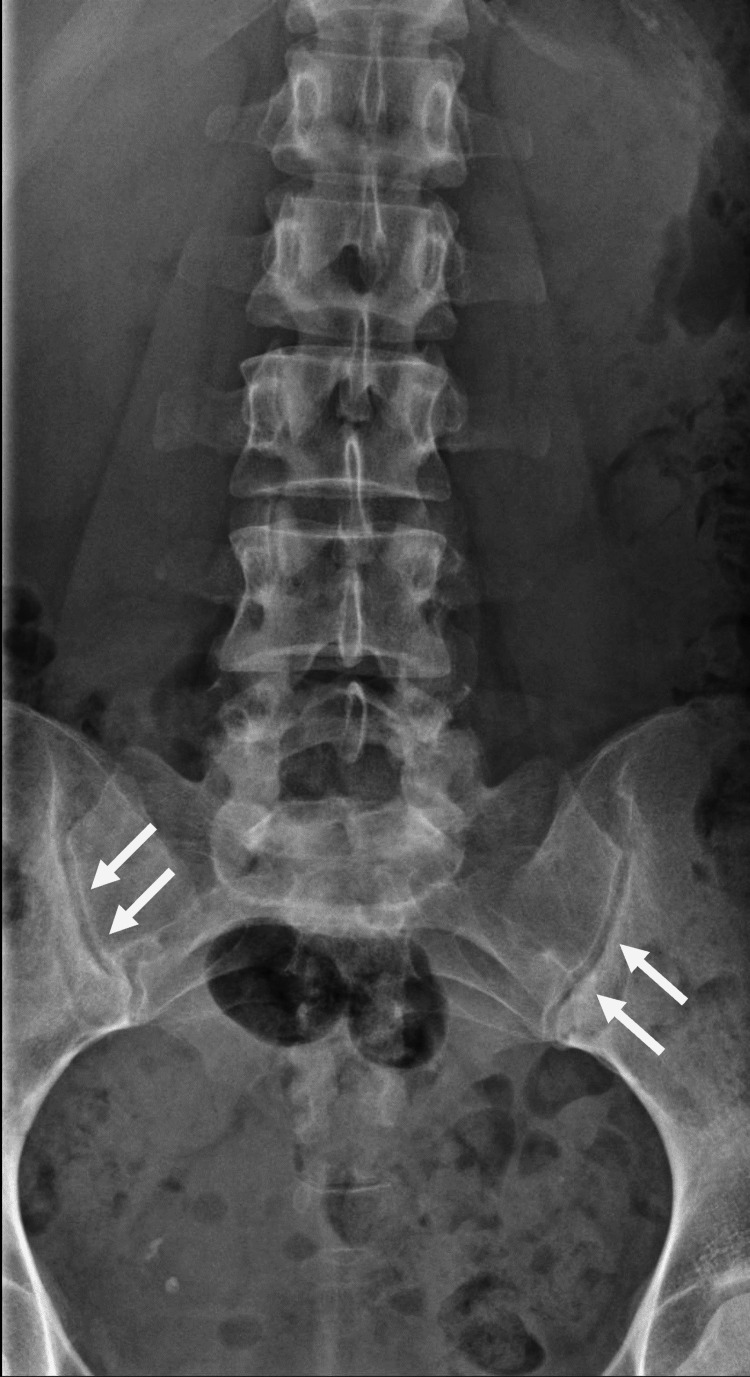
Conventional X-ray Bilateral subchondral sclerosis (arrows) of the sacroiliac joints with discrete erosive changes in posterior-anterior projection. No apparent abnormalities in the lumbar spine.
